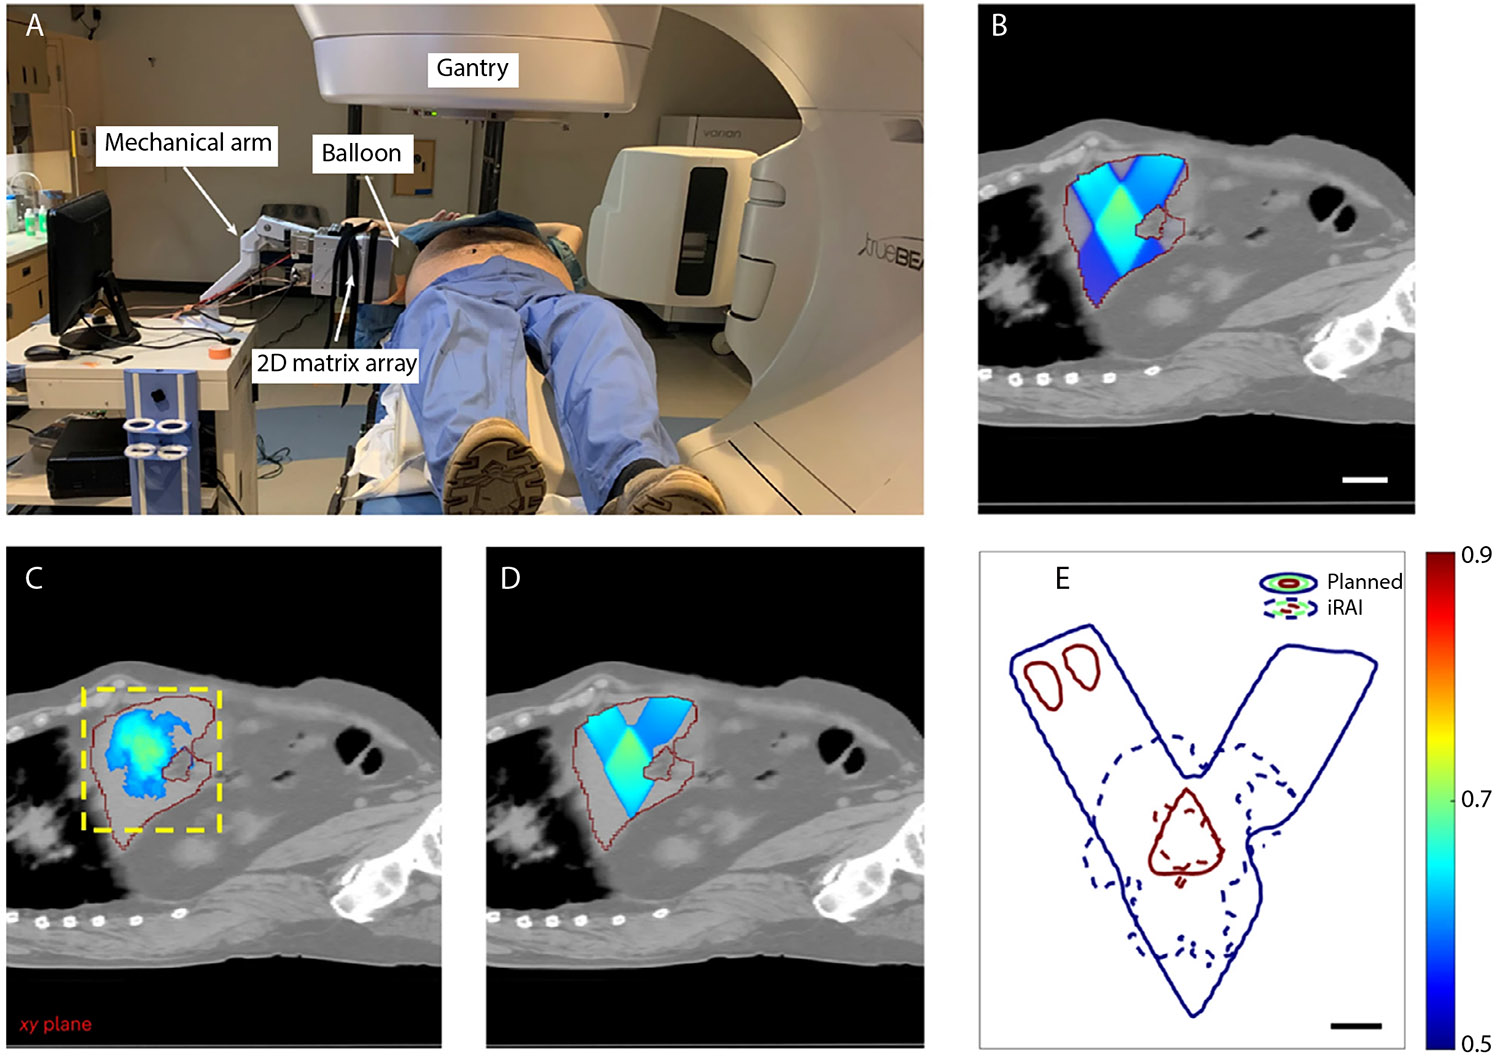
In vivo ionizing radiation-induced acoustic imaging (iRAI) versus the treatment plan on a patient. A, A photograph of the iRAI imaging on a patient taken during radiation therapy. B, The dose distribution of only the 2 static sagittal beams of the treatment plan with a liver mask fused onto the computed tomography scan anatomy structure. Scale bar, 5 cm. C, The iRAI measurement of dose with a liver mask fused onto the computed tomography anatomy structure with the same position as b. The yellow dashed box indicates the field of view of the 2-dimensional matrix array. D, Dose distribution (>50%) of the treatment plan with a liver mask fused on the computed tomography anatomy structure. E, The 50% and 90% isodose lines in the iRAI measurement and the treatment plan. Scale bar, 2 cm. The red line in B to D indicates the boundary of the liver. From ref.^16^
